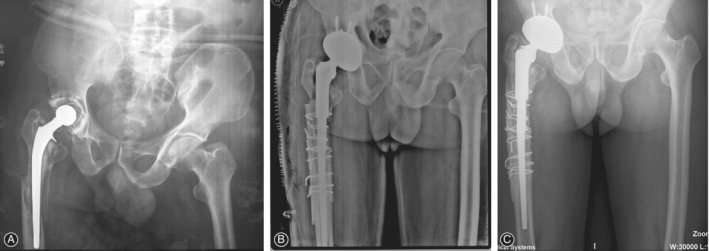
(A) Preoperative anteroposterior (AP) radiograph of the left hip in a patient requiring revision because of aseptic loosening of the cup and periprosthetic fracture of the stem. (B) AP radiograph right after revision arthroplasty with a 64‐mm diameter cementless acetabular component and a modular revision stem. (C) AP radiograph 13.5 years after the index revision.
